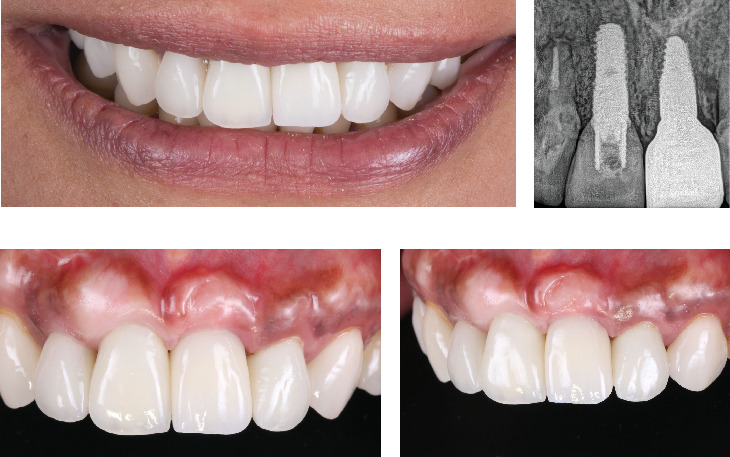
Final restoration in the mouth. (a) Final smile, (b) radiograph, (c) final frontal view, and (d) final left side view.
